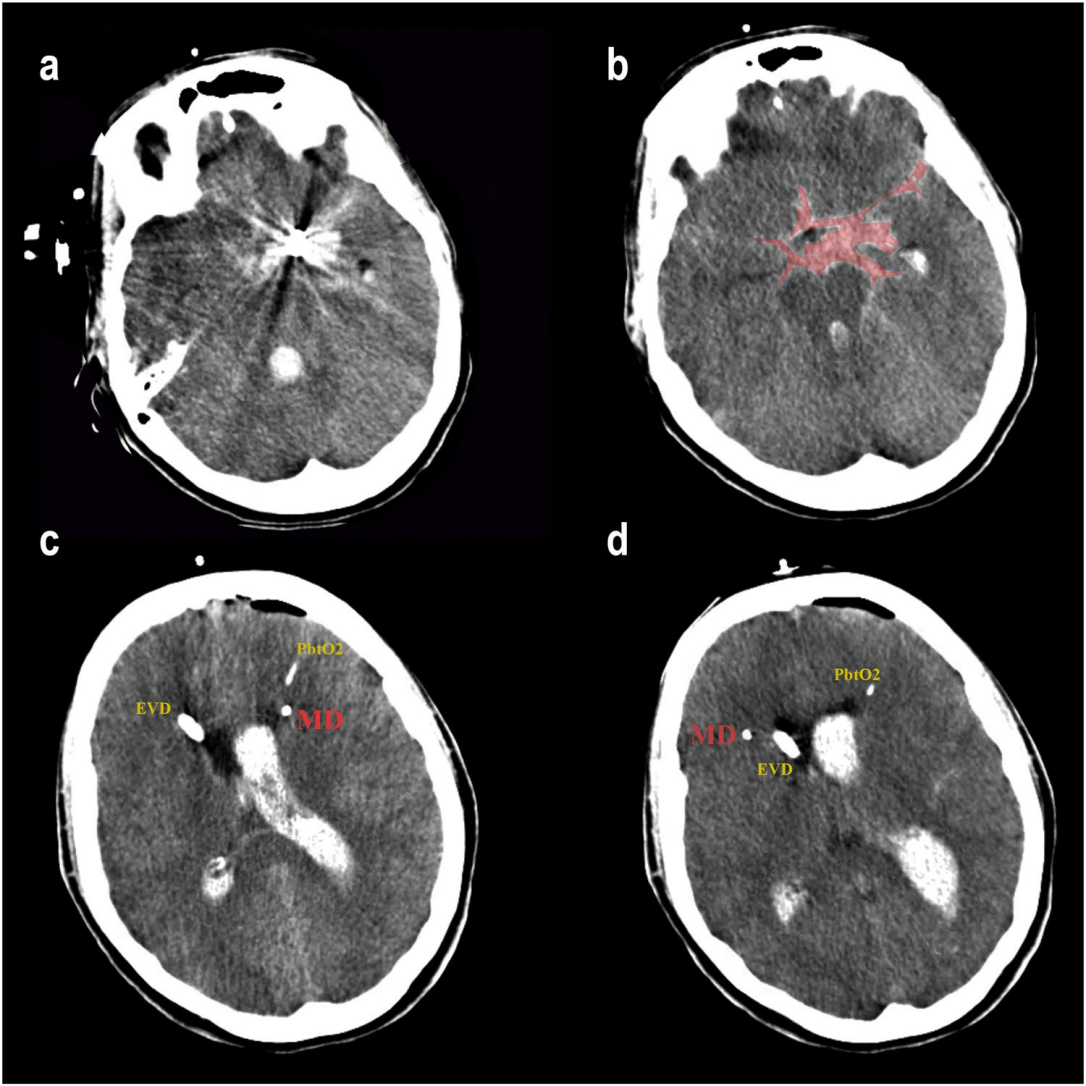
(a,b) Computed tomography of a patient with a poor grade aSAH. Left posterior communicating aneurysm (PCom) embolized. More amount of blood in the left basal cistern of the brain. (c,d) Bilateral monitorization with two CMD probes. An external ventricular drainage placed on the right side and a PbtO2 probe on the left side. The subarachnoid hemorrhage volume is represented by a red shaded area.
